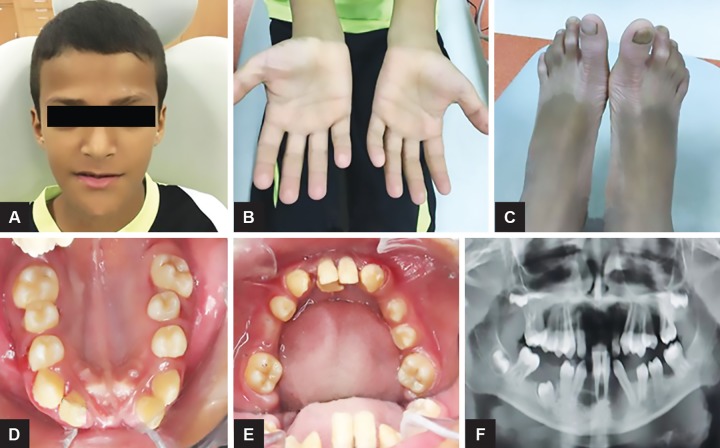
(A) A 12-year-old male patient; (B) palmar hyperkeratosis; (C) plantar hyperkeratosis; (D) intraoral clinical picture of the maxillary arch teeth; (E) intraoral clinical picture of the mandibular arch teeth; (F) orthopantomogram showing multiple loss of teeth and the alveolar bone loss around many present teeth
